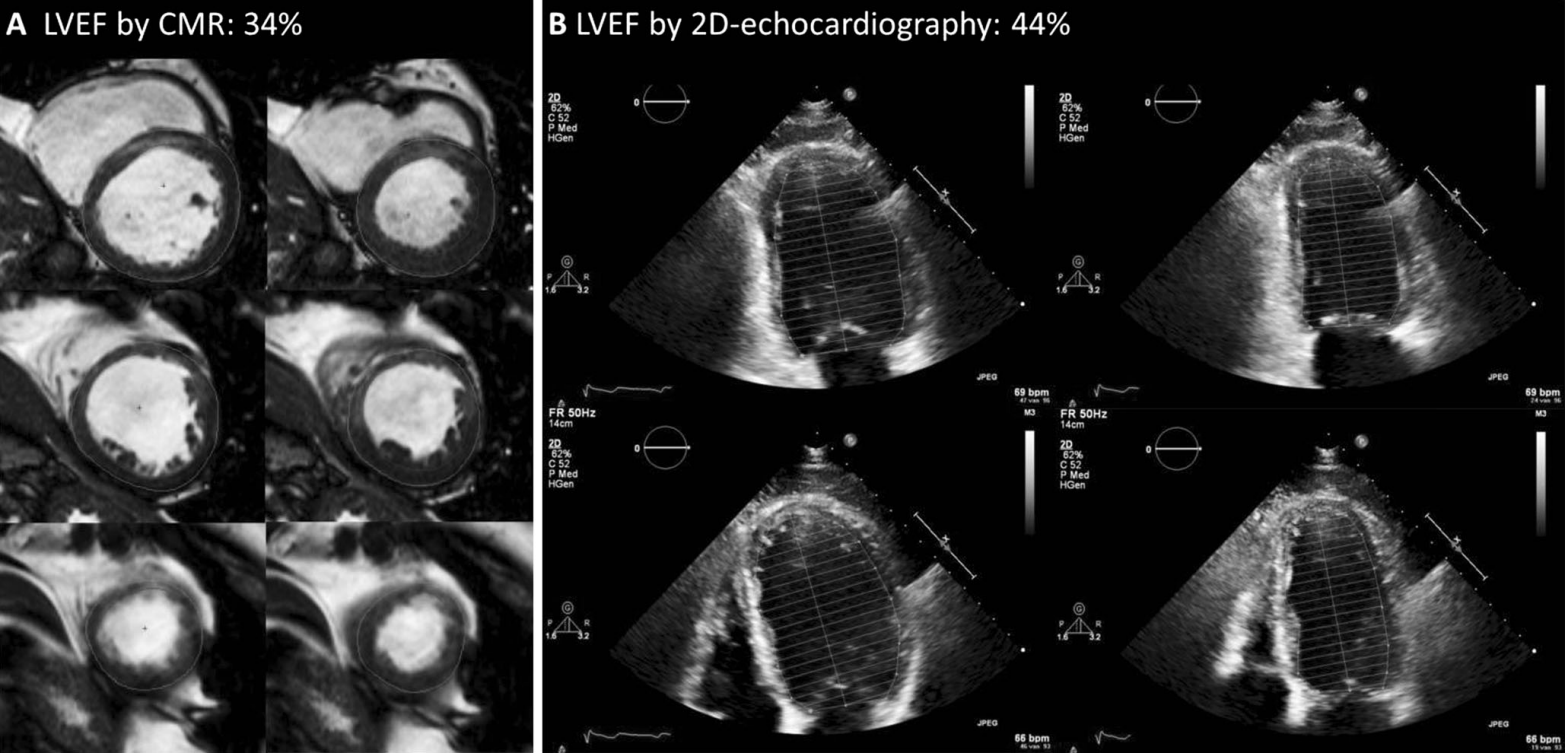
A 67-year-old patient with ischaemic cardiomyopathy who was screened for ICD implantation for primary prevention of sudden cardiac death. No arrhythmias were documented and the patient did not show signs of heart failure. Both 2D echocardiography and CMR were performed, which yielded a difference in LVEF of 10 % (44 vs. 34 %, respectively). Based on echocardiographic analysis, ICD implantation should not have been performed, yet CMR drove the decision to implant the device. To date, 2 years after implantation, no ventricular arrhythmic events have occurred
